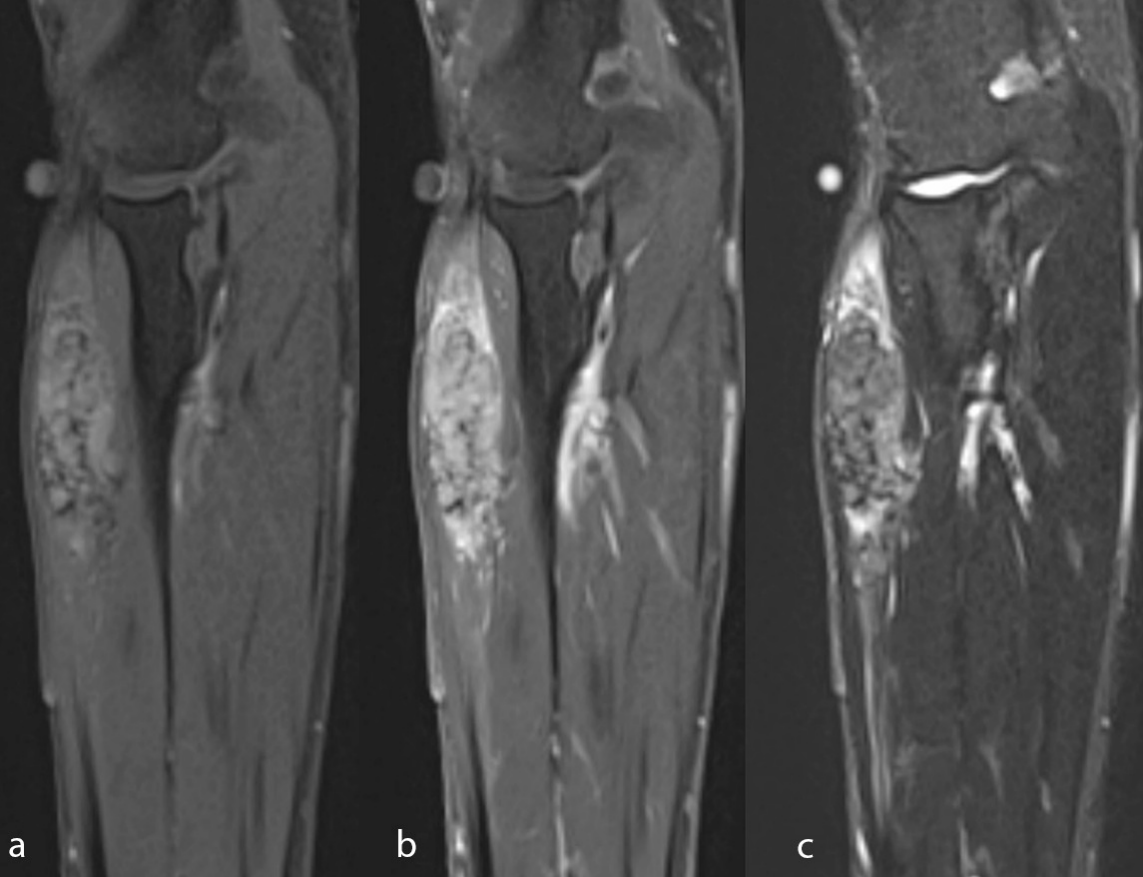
MRI coronal images present pathological mass in the extensor digitorum muscle corresponding to CCRCC metastasis in *T*_1_ weighted fat-saturated pre-contrast image (a) *T*_1_ weighted fat-saturated post-gadolinium image (b) and *T*_2_ weighted fat-saturated image (c) respectively.
